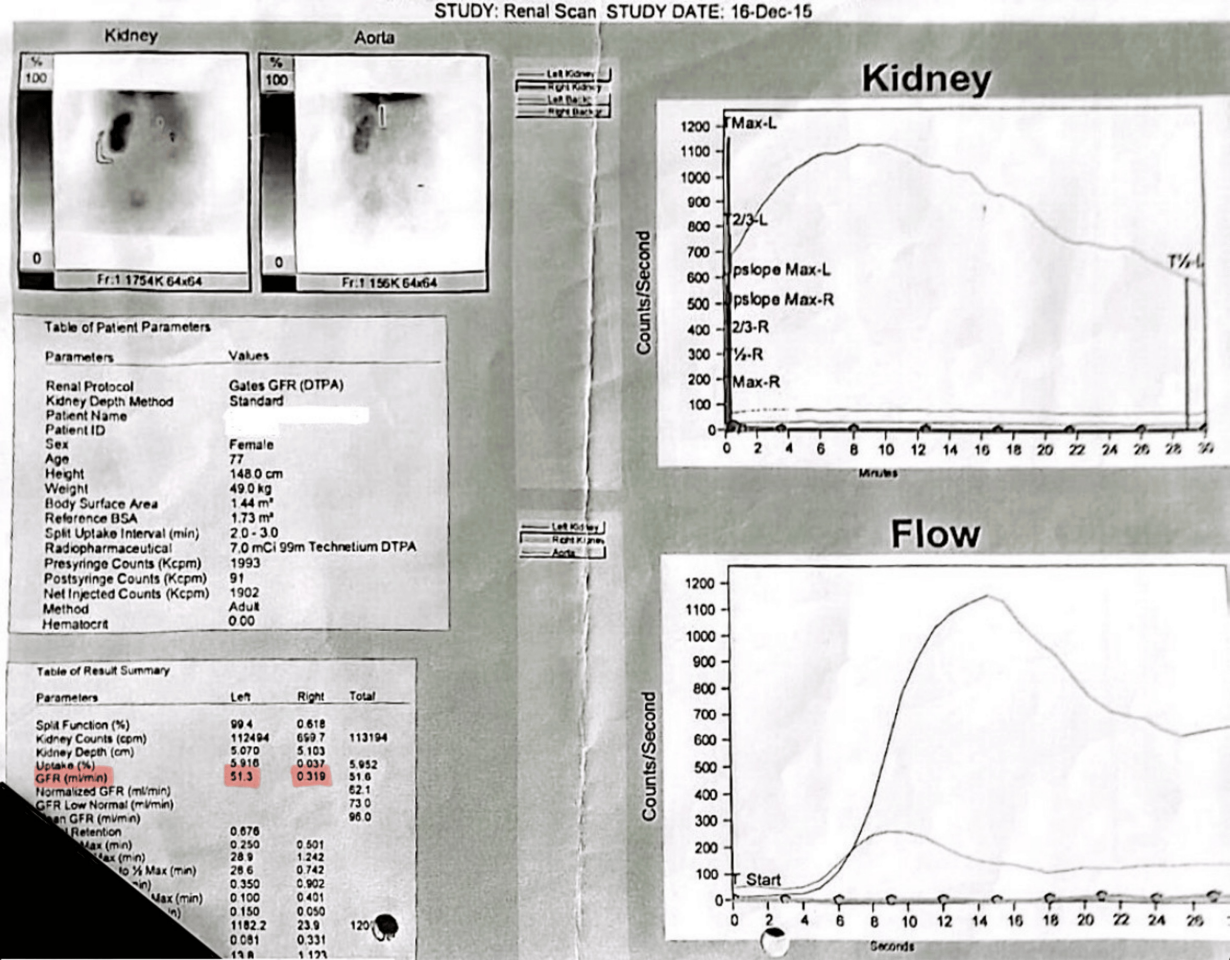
Diethylenetriaminepentaacetic acid (DTPA) scan of the patient performed in 2015. 99mTc-DTPA renal scintigraphy, which shows differential renal function. The graphs represent the tracer uptake and flow through the two kidneys, with the right kidney forming a flat curve representing poor renal function. The table in the lower left provides the exact functional data for each kidney, and GFR values are highlighted in red.
